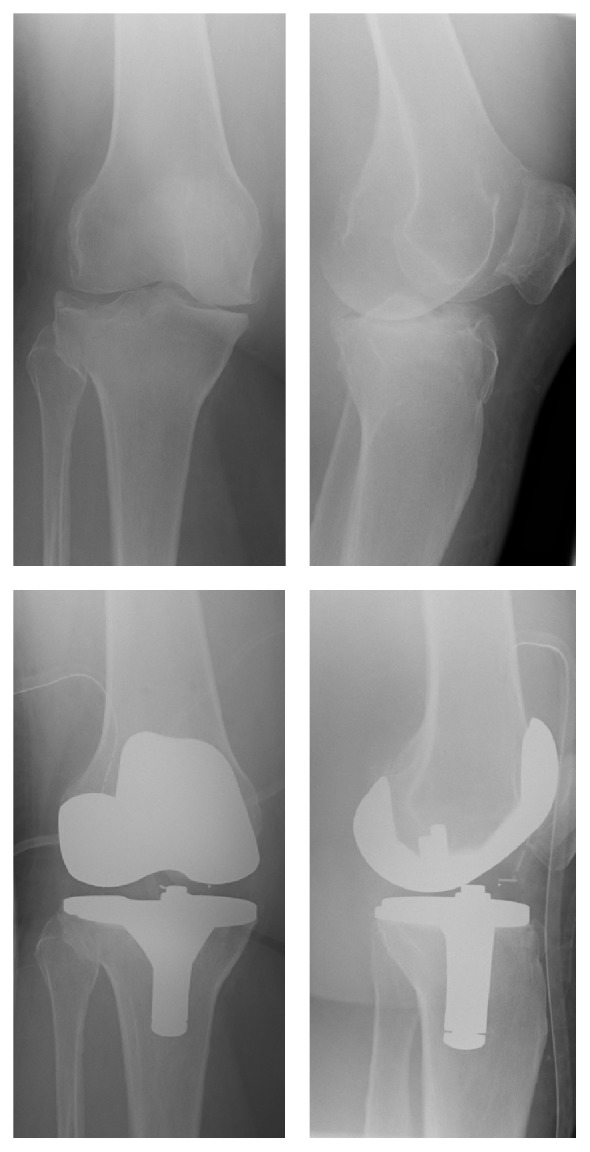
Female, age 83, tibia fracture AO 41B3, e.motion (Braun), postoperative X-ray, KSS1 83, KSS2 80, and WOMAC 87 (12th month's follow-up).
